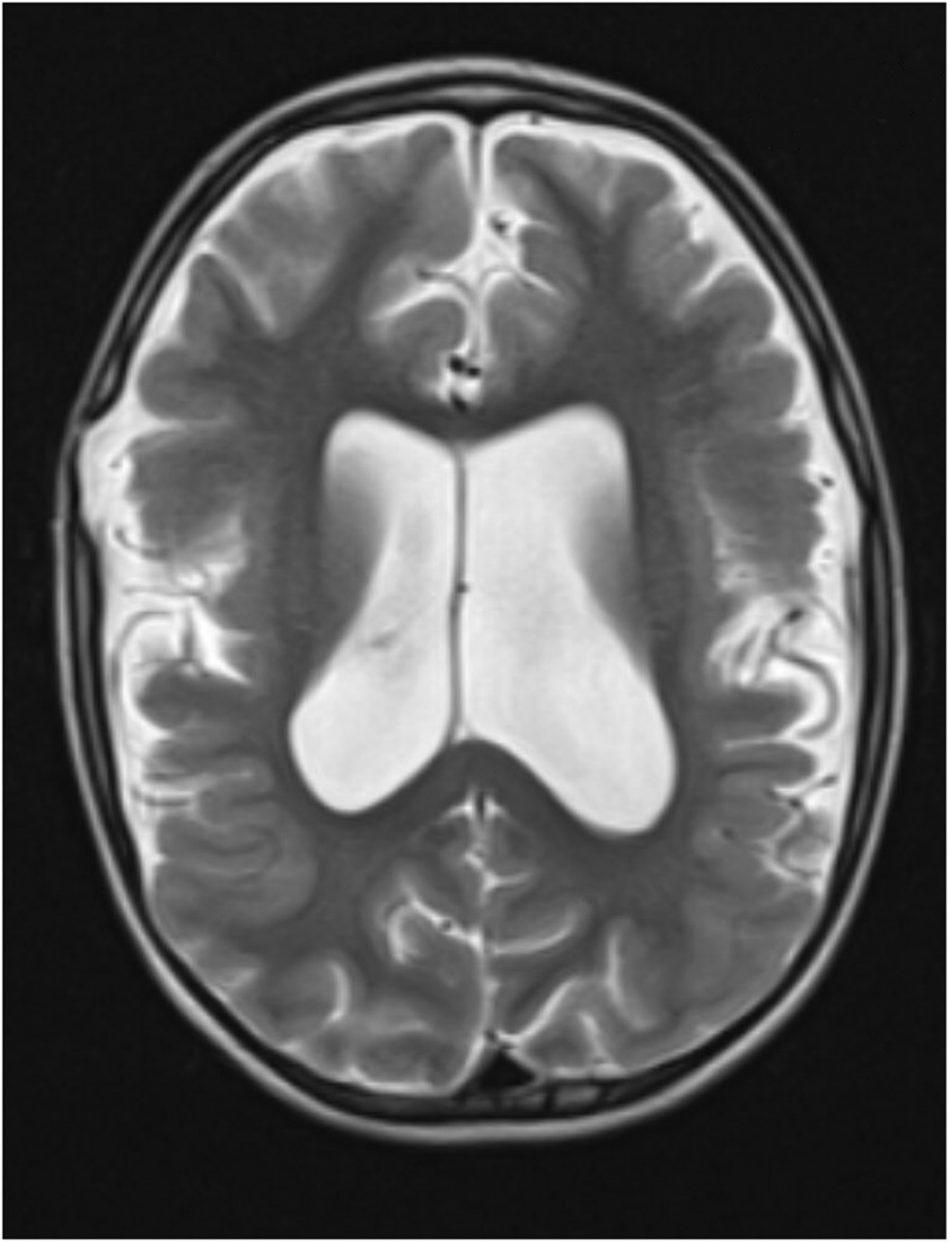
Magnetic resonance imaging of patients suffering from dysmyelination and seizures.
